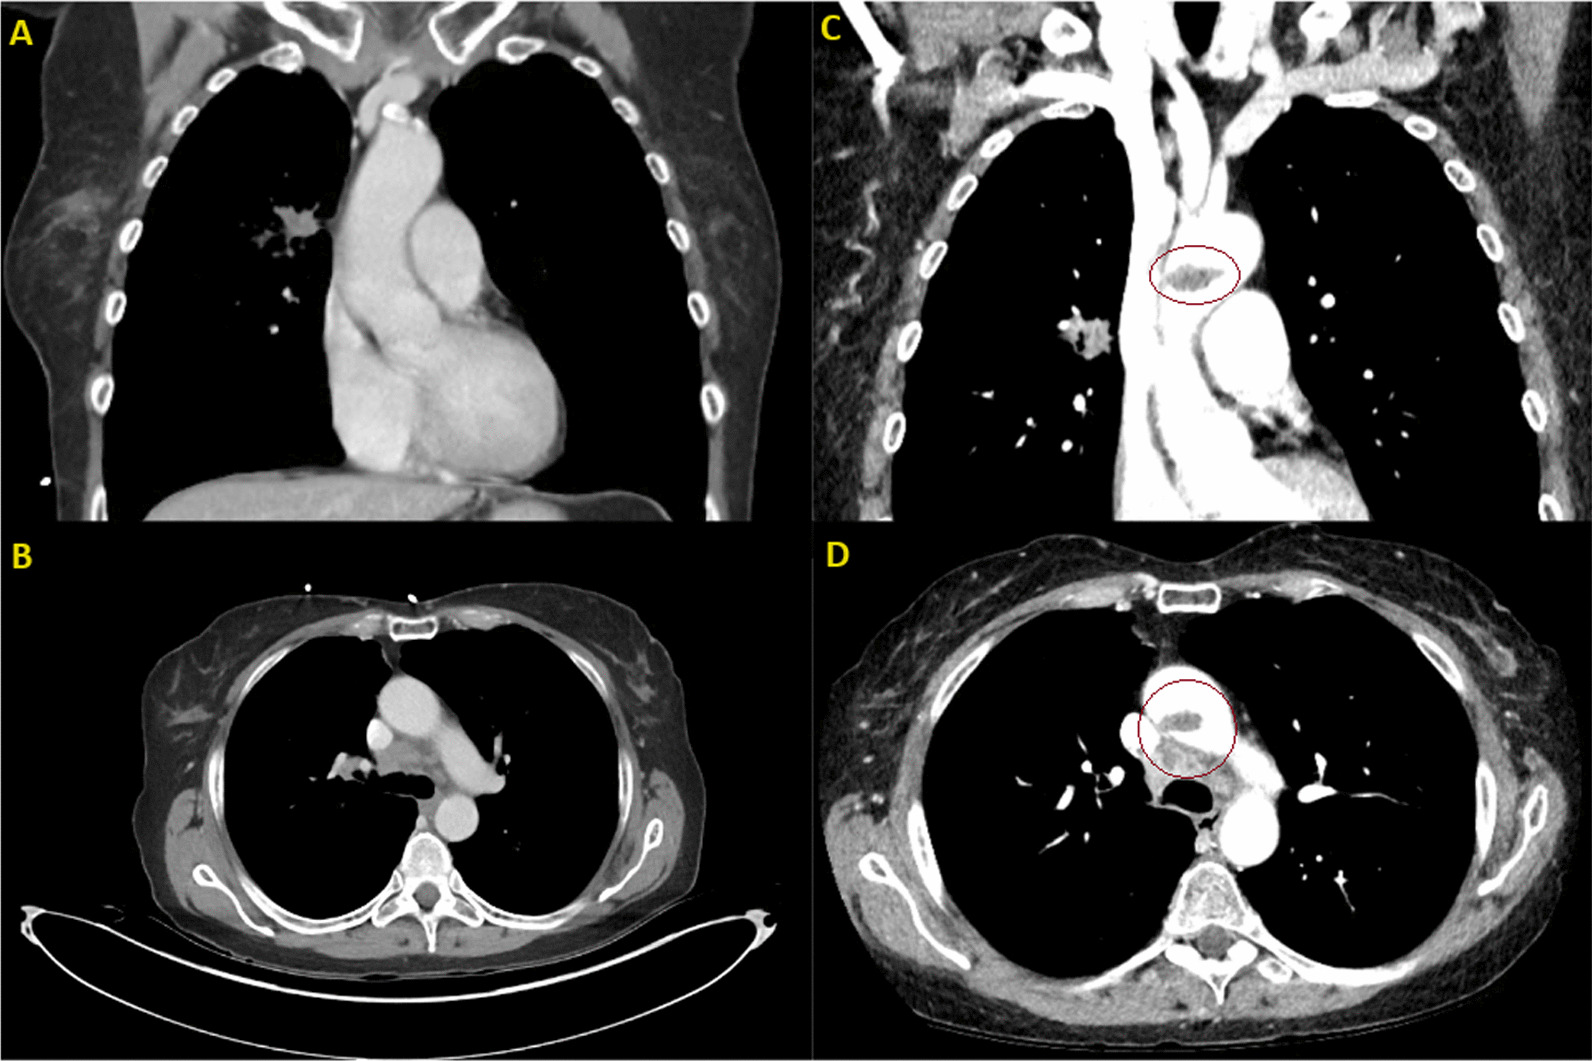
A and B Coronal and axial sections of the initial contrast enhanced computed tomography of the chest, respectively (from Fig. 2), failing to demonstrate aortic pathology, including dissection or aneurysm. There is calcification noted at the ostia of the left common carotid and left subclavian, but negligent atherosclerosis in the ascending aorta. C and D Comparison of coronal and axial slices, respectively, this time from a computed tomography angiogram of the chest, demonstrating the new aortic thrombus proximal to the aortic arch
